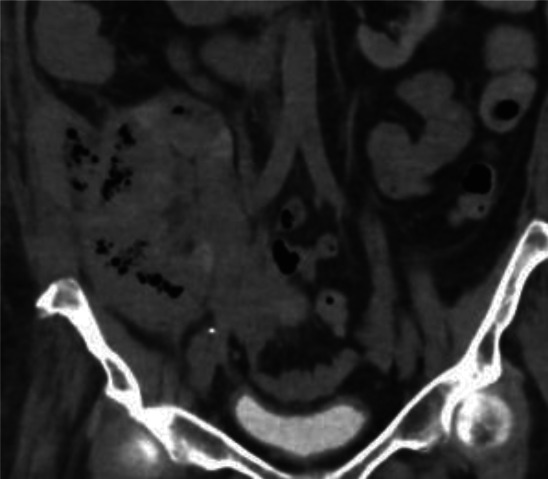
CT of the abdomen without contrast 1 month later demonstrates interval improved foci of air within the transplant kidney. There is perinephric fluid collection concerning for abscess.
